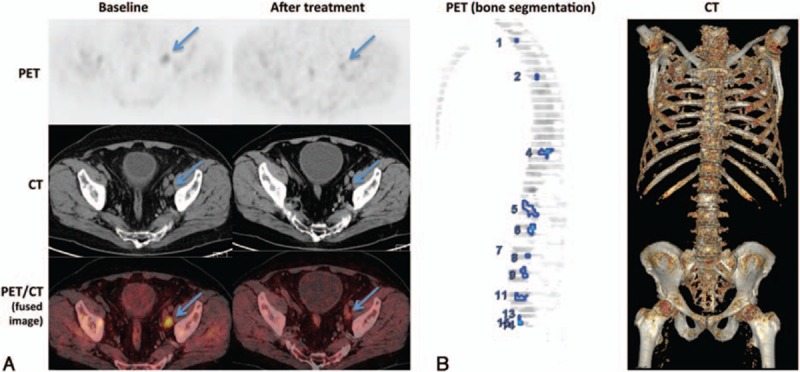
(A) PET/CT scans of a metastatic iliac lymph node before and after 3 months of treatment with abiraterone acetate. (B) Calculation of a bone lesion index by measuring the PET uptake volume with an automated segmentation algorithm and dividing this volume by the volume of the entire skeleton as determined by CT. PET/CT = positron emission tomography/computed tomography.
